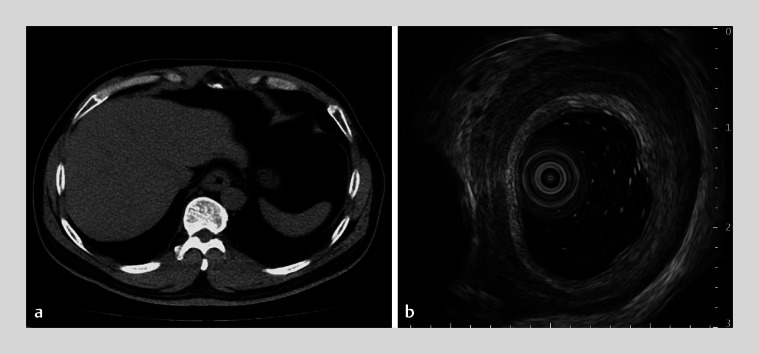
Computed tomography and endosonographic findings of the esophagus.
